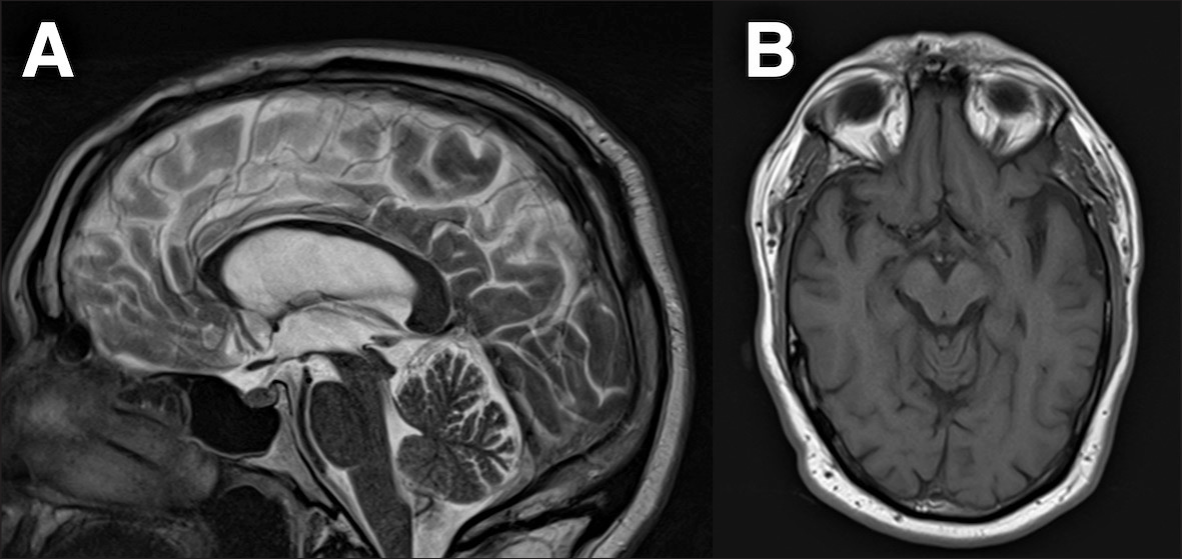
A: Sagittal T2-weighted magnetic resonance imaging taken at the time of clinical presentation shows no evidence of hummingbird sign. B: Axial T1-weighted MR at the same time point does not show midbrain atrophy, anteroposterior midbrain diameter = 15 mm.
